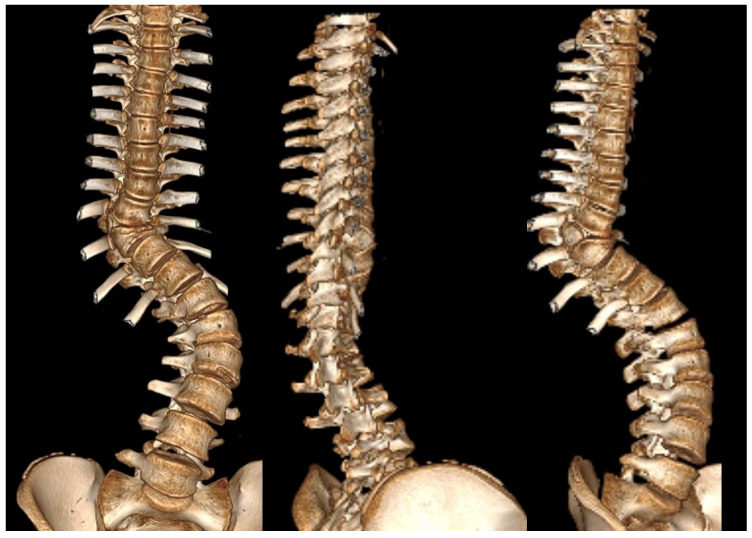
3D CT of 17-year-old boy with congenital scoliosis and insulin-treated diabetes, who underwent P-VCR T10 (posterior-only vertebral column resection of T10).
